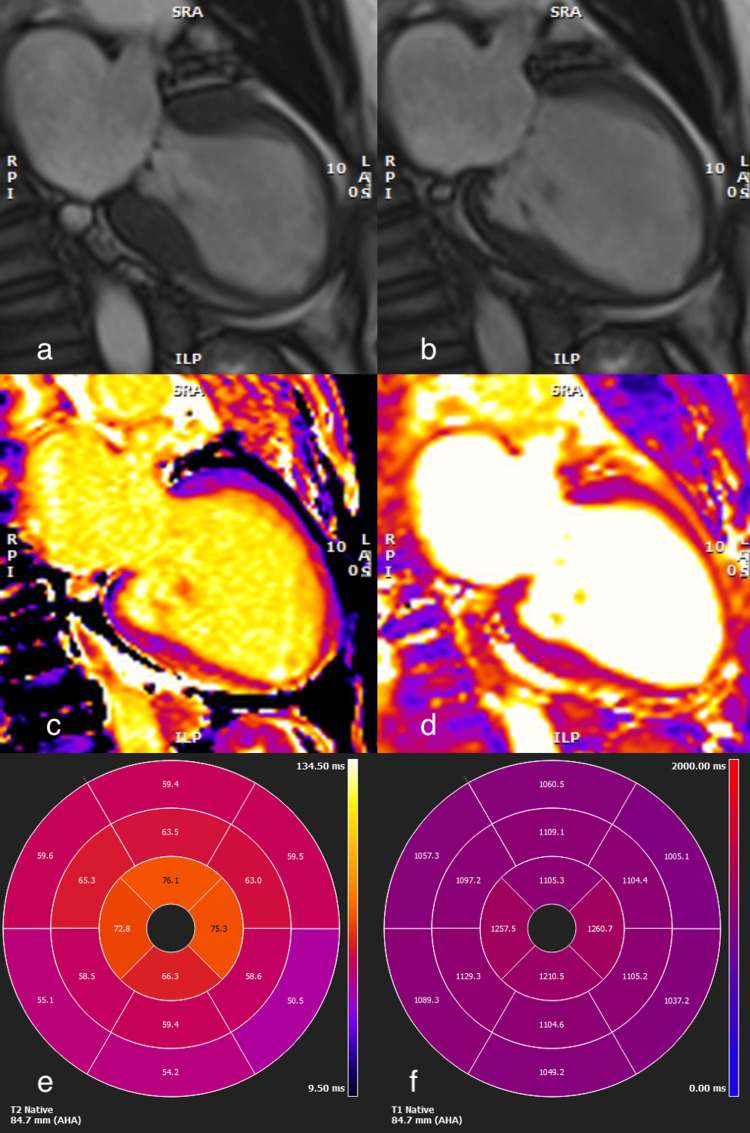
Cardiac MRI images showing dilated LV in Takotsubo-like pattern a) 2-chamber systole, b) 2-chamber diastole, c) T1 2-chamber, d) T2 2-chamber. Bullseye plots showing 16 segment distribution of e) T2 and f) T1 values, with a typical distribution as seen in Takotsubo (elevated from mid ventricle to apical levels). LV: Left ventricular
